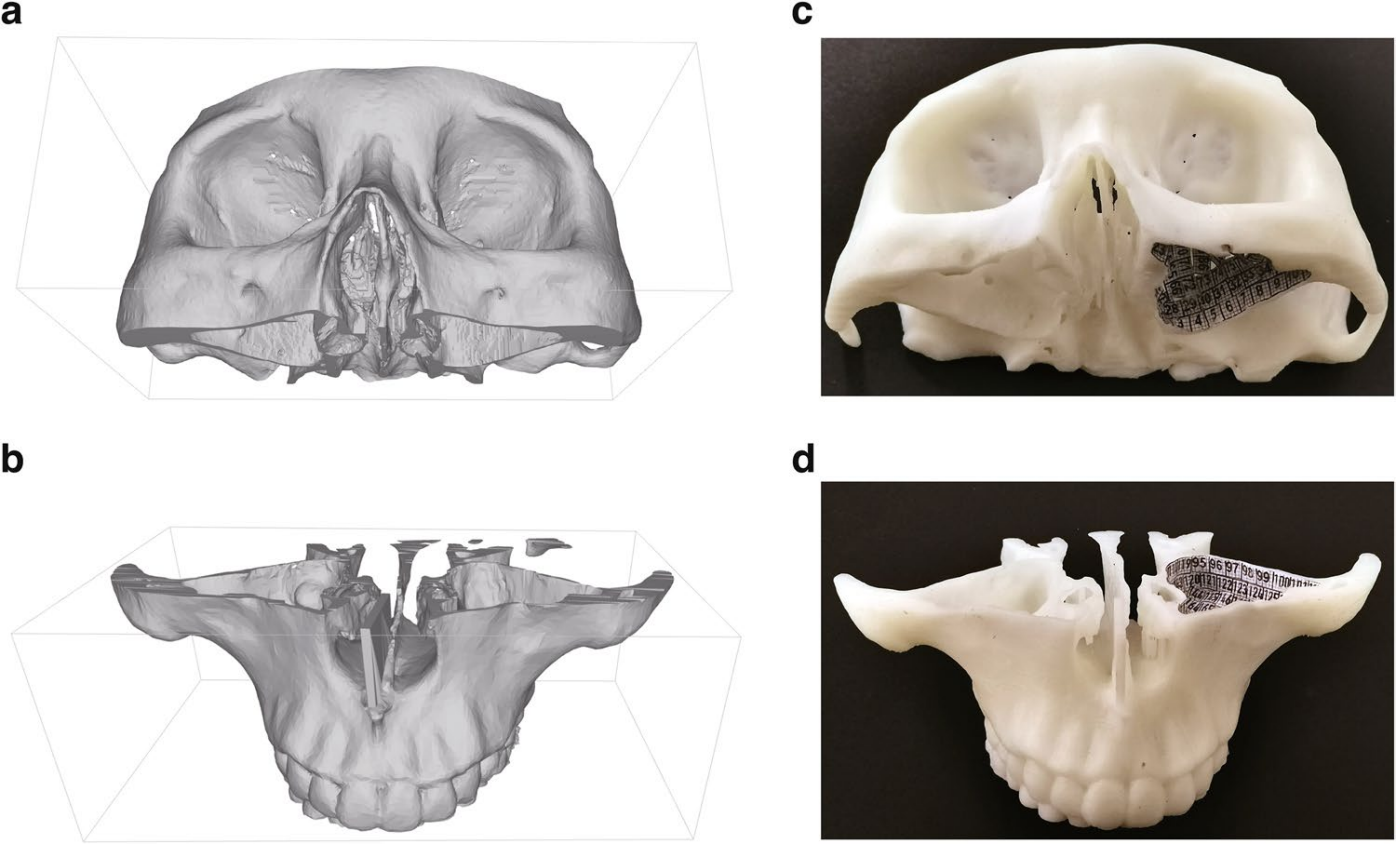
The 3D-printed model of MS. a, b The bone structure was reconstructed by CT image of paranasal sinus from a healthy volunteer. It was horizontally divided into upper (a) and lower (b) parts from the plane below the natural ostium of MS. c, d 3D-printed model was used in the following endoscopy. Marked grid papers were stuck to the inner surface of the left MS
